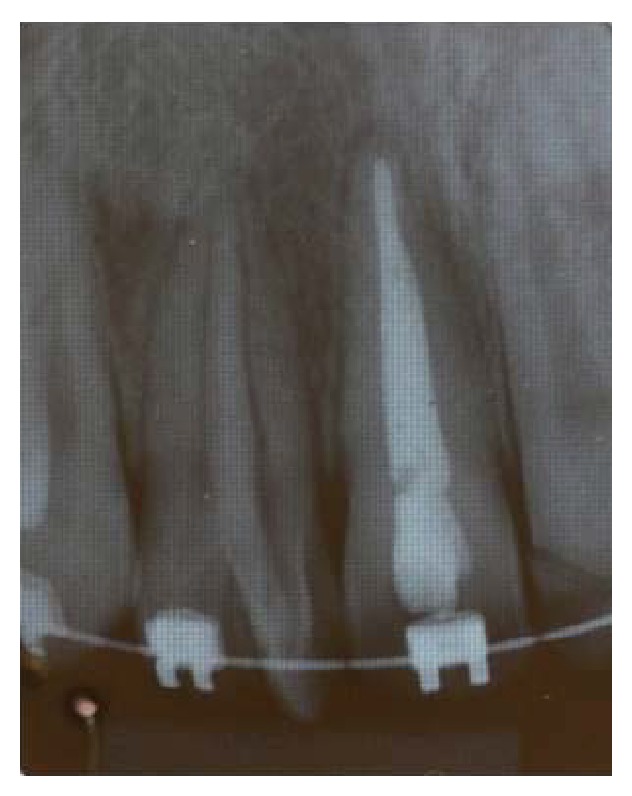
Periapical radiograph of the right maxillary lateral incisor and the supernumerary tooth with increased radiopacity in both roots suggesting overlapping image.
